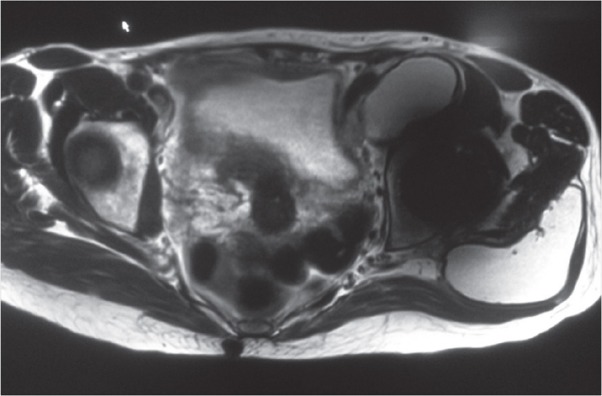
MRI of case 30, showing large fluid-filled pseudotumor.
